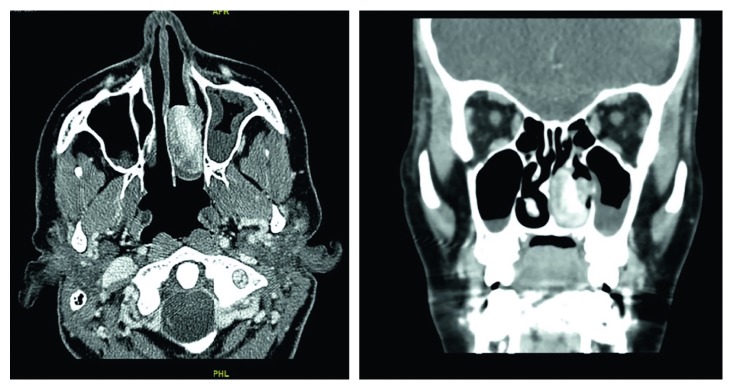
Axial and coronal sinus CT scans with contrast showing a heterogeneously enhanced mass involving the middle and lower meatus of the left nasal cavity with normal adjacent bony structures. In its maximum dimensions, the mass measured 3.2 × 2.2 × 1.5 cm.
